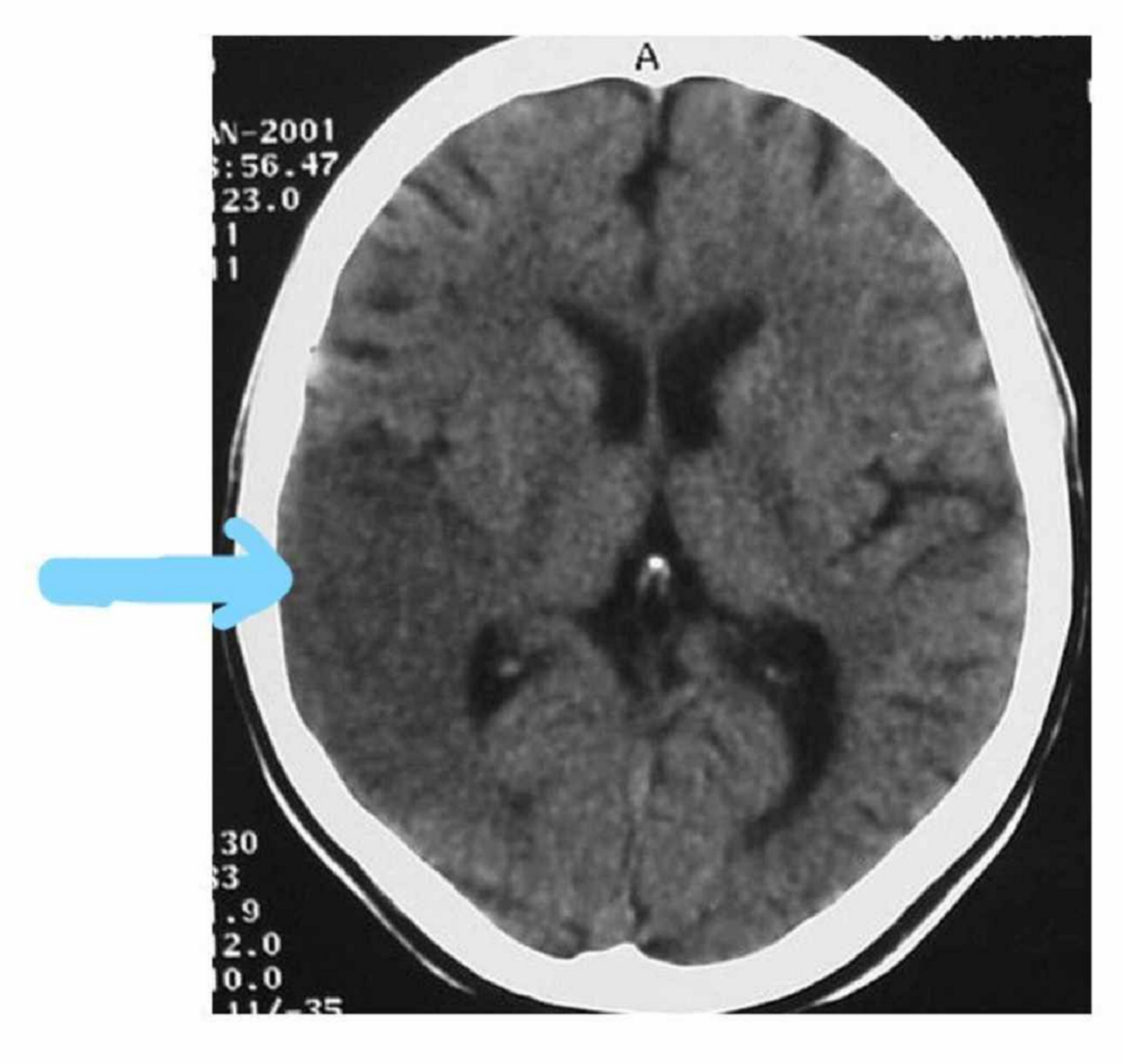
Brain CT with hypodense area compatible with right temporo-parietal ischemia CT: computed tomography
